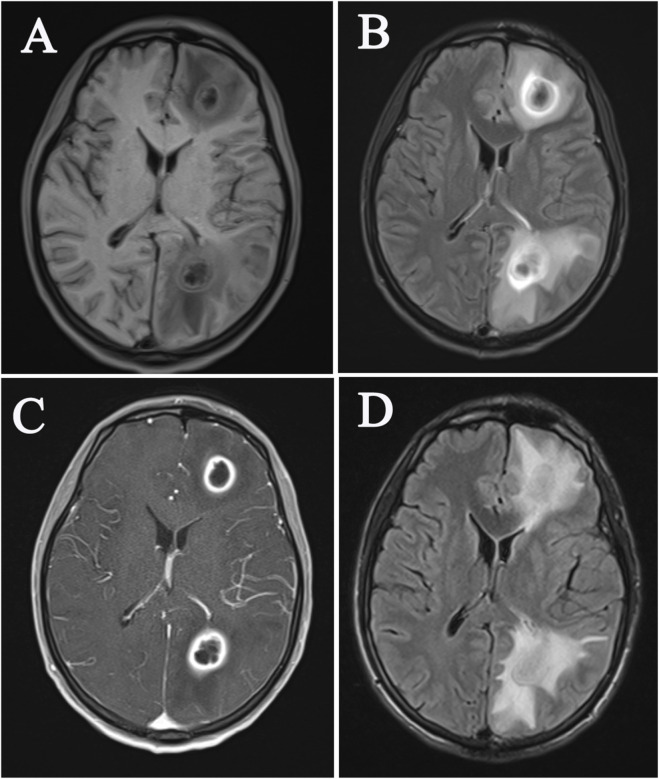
Brain MRI imaging features of patients at initial diagnosis (A-C) and after a follow-up examination (D).
